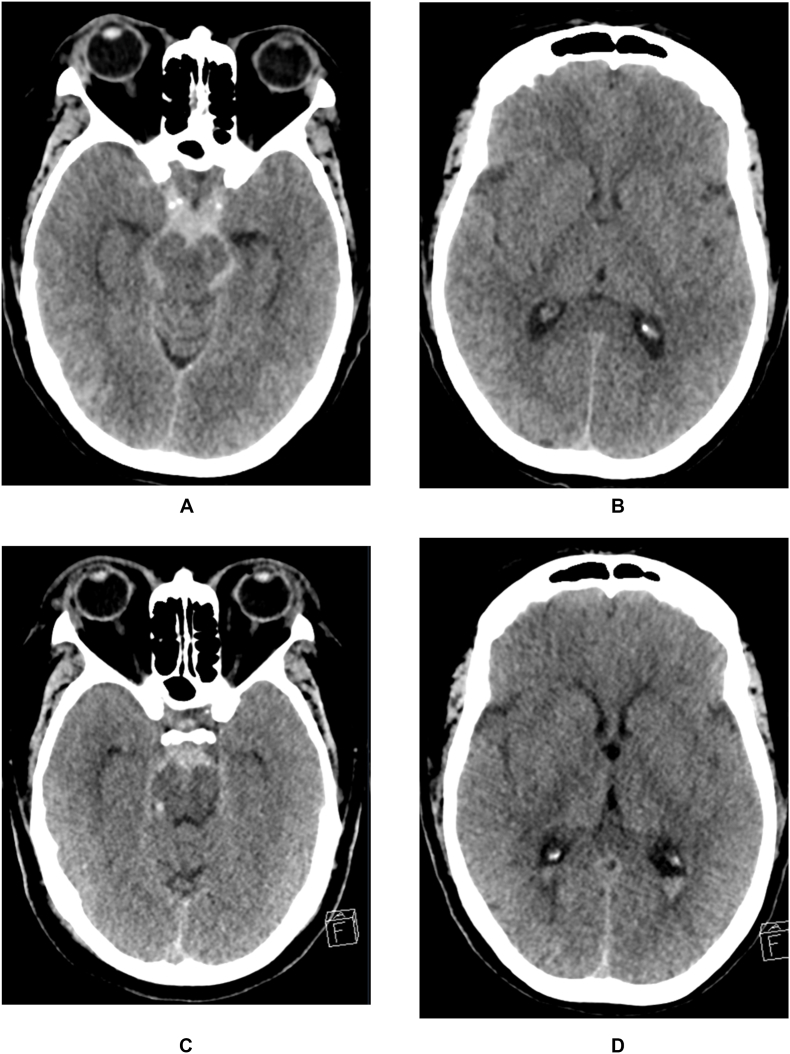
56-year-old female patient with non-aneurysmal perimesencephalic subarachnoid hemorrhage (PMSAH). On the initial non-contrast CT (NCCT) scan a significant amount of blood is seen in the suprasellar and ambient cistern (A), but absent intraventricular blood (B). On the repeat NCCT 34 h later, the amount of blood in the suprasellar cistern has decreased (C) with now presence of intraventricular blood (D).
